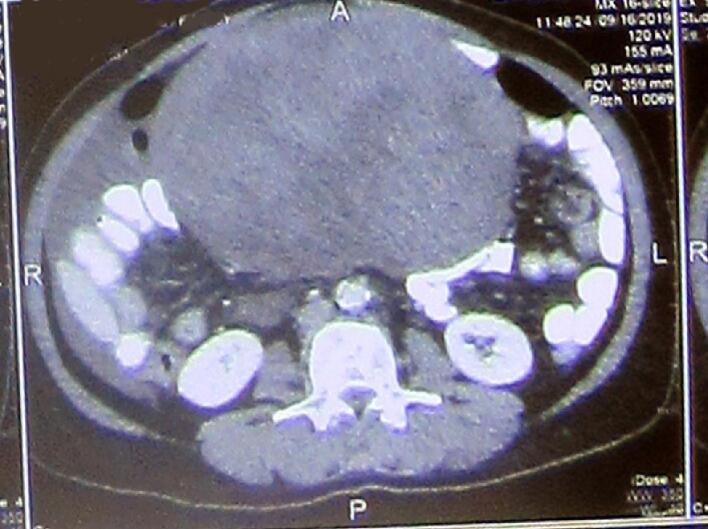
Abdomino-pelvic CT scan, horizontal view.
